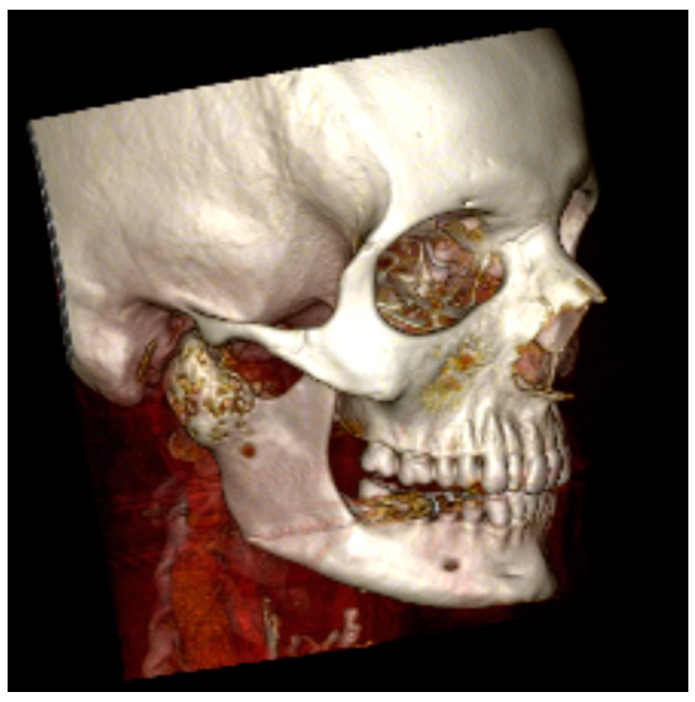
Computed tomography of the peroperative presentation, showing an expansive lesion of the right mandibular condyle.
